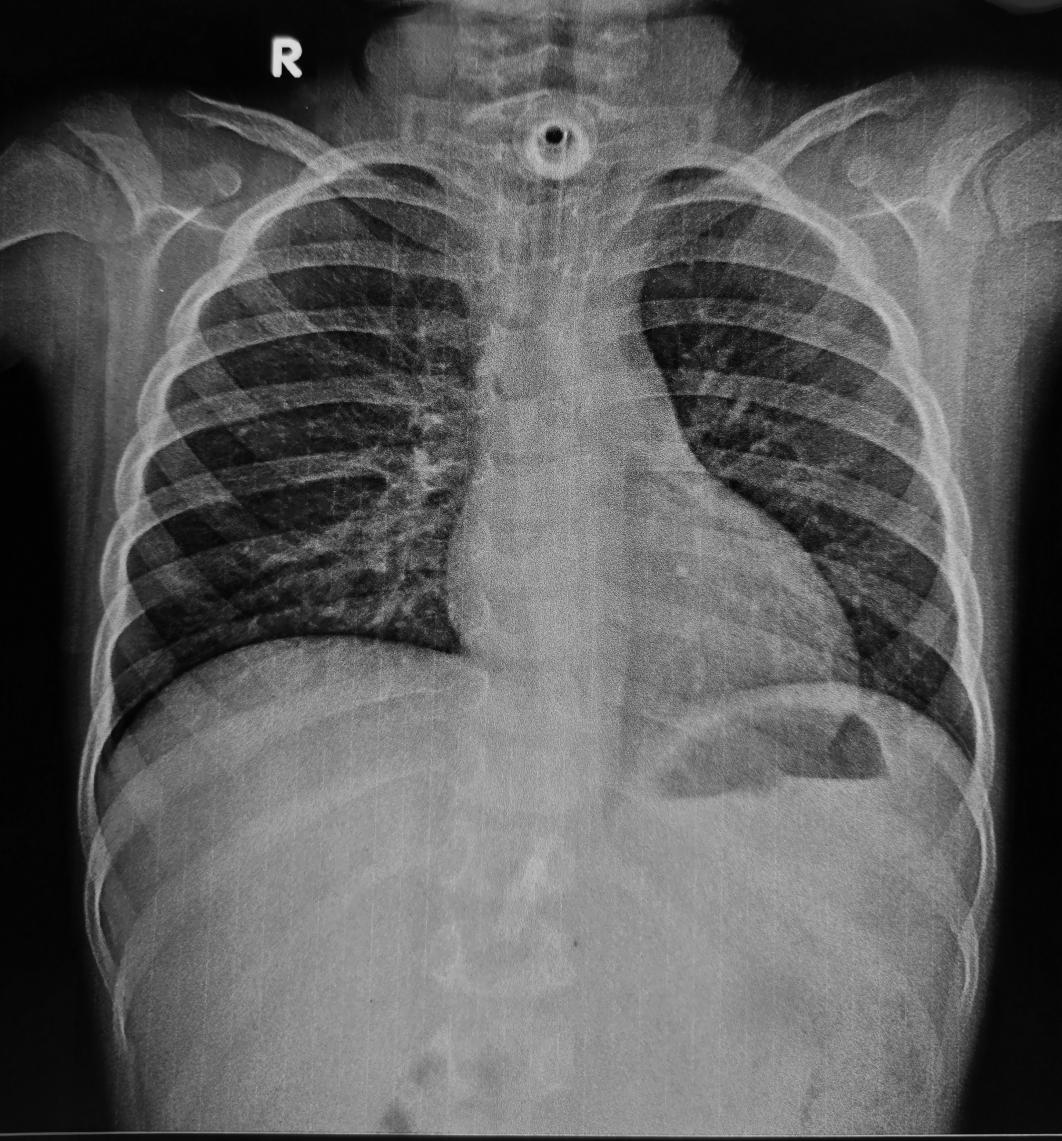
Chest X-ray of the patient before tracheoplasty.
